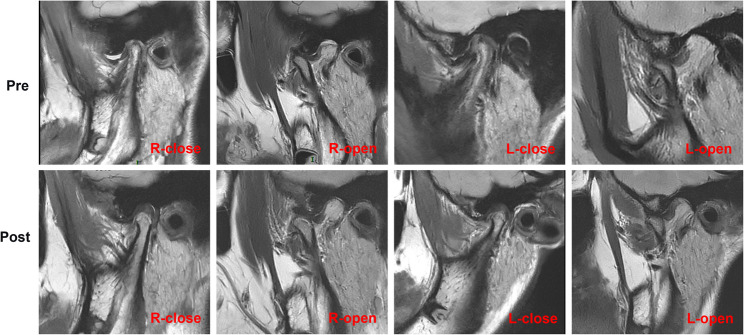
Pre- and post-treatment magnetic resonance imaging (MRI) of a representative patient. Pre: before treatment; Post: after treatment; R-close: right temporomandibular joint (TMJ), closed-mouth position; L-close: left TMJ, closed-mouth position; R-open: right TMJ, open-mouth position; L-open: left TMJ, open-mouth position
